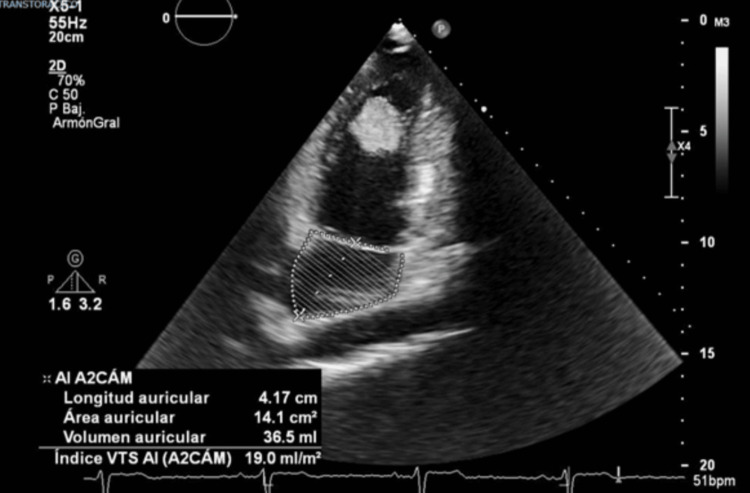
Apical two-chamber view showing a large, mobile, pedunculated 23 mm mass within the left ventricle, attached to the interventricular septum.
